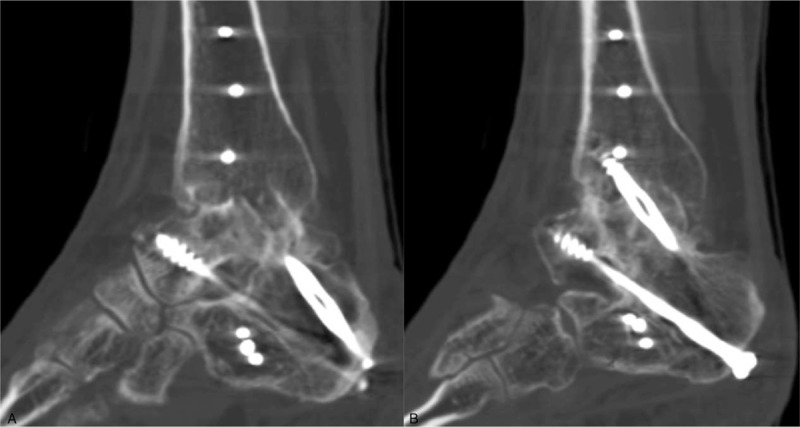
The CT scan taken at 4-month follow-up after surgery demonstrated bone union and a good alignment of the foot.
